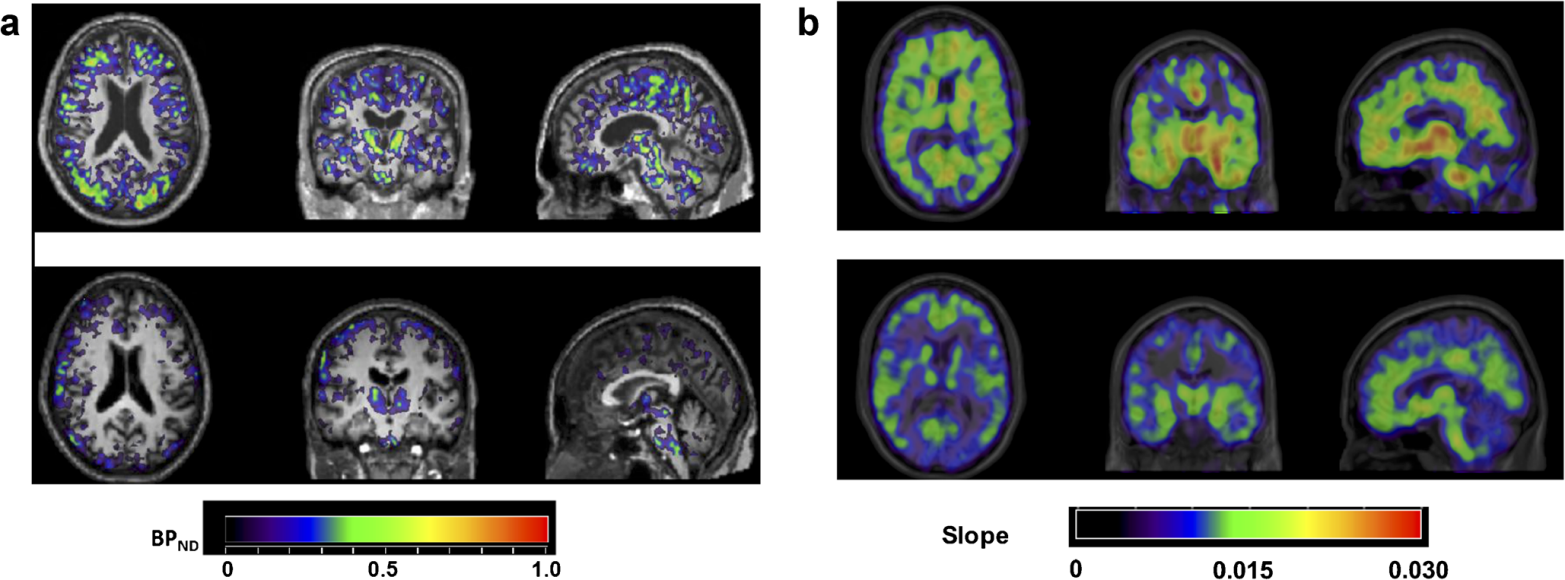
a Parametric ^11^C-*(R)*-PK11195 PET images of microglial activation in one MCI patient (upper row) and one healthy volunteer (lower row); PET images are displayed on each subject’s respective T1-weighted MRI scan normalised to the SPM5 T1 brain template and the colour bar indicates non-displaceable binding potential (BPND) values. b Parametric ^11^C-deuterium-L-deprenyl PET images of astrocyte activation in one Aβ-positive MCI patient (upper row) and one healthy volunteer (lower row); PET images are displayed on each subject’s respective T1-weighted MRI scan normalised to the SPM5 T1 brain template and the colour bar indicates modified-reference (cerebellar grey matter) Patlak slope values; the ^11^C-deuterium-L-deprenyl PET imaging data were kindly provided by Prof. Agneta Nordberg, Karolinska Institutet, Sweden
